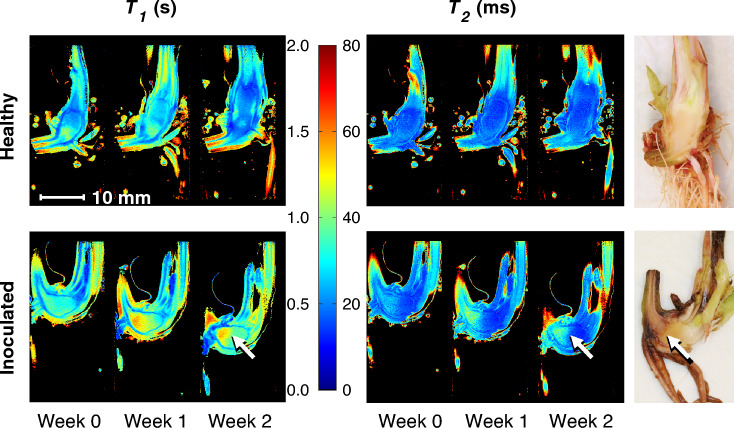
Examples of relaxation time maps of healthy and inoculated plants during development. Quantitative $$T_{1}$$ and $$T_{2}$$ relaxation time maps of healthy and inoculated strawberry crowns during a two-week development period after inoculation. $$T_{1}$$ and $$T_{2}$$ relaxation time maps are calculated from fast spin echo multi slice—inversion recovery (FSEMS-IR) and multi echo multi slice (MEMS) pulse sequence MRI data, respectively. Differences in the visibility of the stolon and root structures between time points is due to slightly different MR image slice positions. The representative examples here are #4 from healthy and #1 from inoculated plants presented in Supplementary Fig. S1 online. Relaxation time data from surrounding water have been omitted (black). The white arrow indicates the observed progressing edge of the infection in the dissection photograph as well as the same position at the relaxation time maps. The color gradient represents the relaxation times for each region, from relatively low relaxation time values (blue) to relatively high relaxation time values (red), and relaxation time values in-between (cyan, green, yellow and orange).
